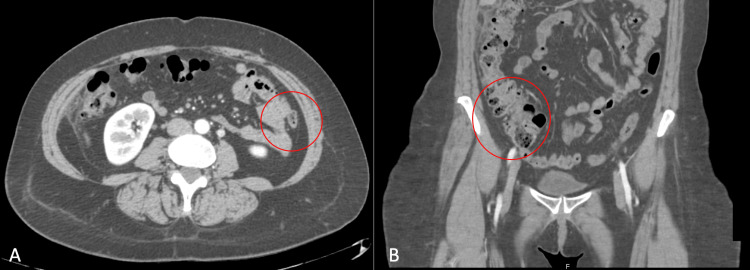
CT scan of abdomen pelvis with and without contrast. A. Transverse view and B. Sagittal view with red circles indicating area of epiploic appendagitis on ascending colon, with surrounding inflammatory fat stranding, and thickening of the adjacent peritoneum. CT: computerized tomography
